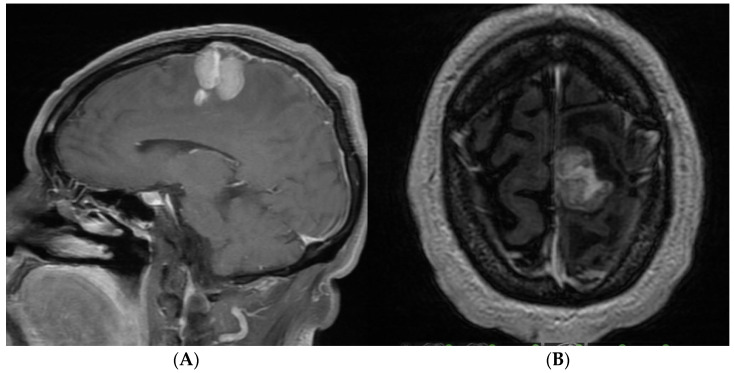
(A) Brain MRI sagittal view with homogeneous oval image, multilobulated 1.3 × 2.53 × 3.49 cm. (B) Brain MRI axial view with homogeneous oval image, multilobulated 1.3 × 2.53 × 3.49 cm.
